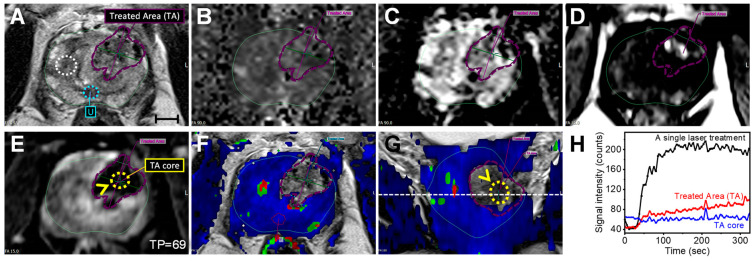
AuroLase^®^ Therapy assessment with mpMRI on Day 5. (A–E) The treated tumor area (dashed purple line) had heterogeneous and low signal intensities on an axial *T*_2_w MRI (A), low signal intensities on an axial high b-value DWI (B), low ADC signals on an axial ADC map based on DWI with b-values of 50 and 800 s/mm^2^ (C), high intensity on an axial *T*_1_w MRI without background subtraction and before *T*_1_ contrast agent injection (D), and absent signal enhancement on an axial background-subtracted *T*_1_w MRI, the last *T*_1_w MRI acquired (TP = 69) during the DCE sequence acquisition (E). (F) An axial *T*_2_w MRI with a DCE (contrast agent wash-in/out) color map overlay corresponds to the dashed white line across the coronal plane in (G). The two planes highlight the treated area with a void space within the prostate gland capsule. (H) A CA signal enhancement kinetic at three regions of interest: (1) the treated tumor (red line), (2) its core (blue line), and (3) a single laser treatment at the right side of the prostate gland (black line) corresponds to the dashed white circle in (A). Additional color coding: solid green line—the prostate capsule, yellow arrowhead/dashed circle—the treated tumor core, and cyan dashed circle—the urethra (U). Note: the treated area was manually created on the DCE color map and was propagated to the rest of the images for further analysis (scale bar = 10 mm).
